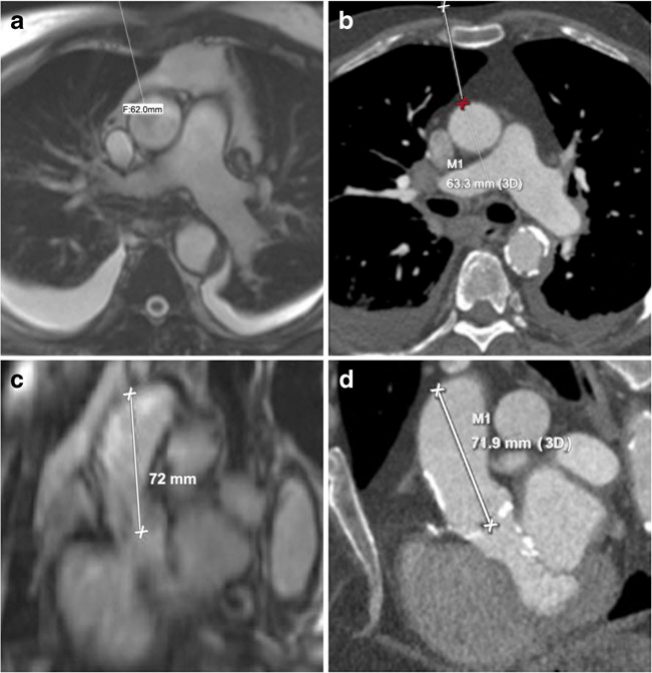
Identification of access site in tubular ascending aortic for transaortic implantation on MRI. Skin to aortic distance measured on axial non-gated SSFP sequence (a) similar to CTA (b). Access site on aorta to annular plane distance measured from multiplanar sagittal oblique reformats obtained from the same axial non-gated SSFP sequence (c). Same measurement in this patient obtained from CTA (d)
